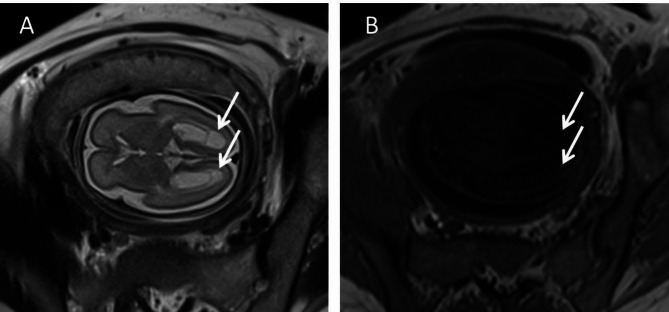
Prenatal MRI of fetal brain. (A) and (B) T2 and T1 weighted imaging, respectively. Images show subependymal cysts in the posterior horn of bilateral lateral ventricles (arrows)
